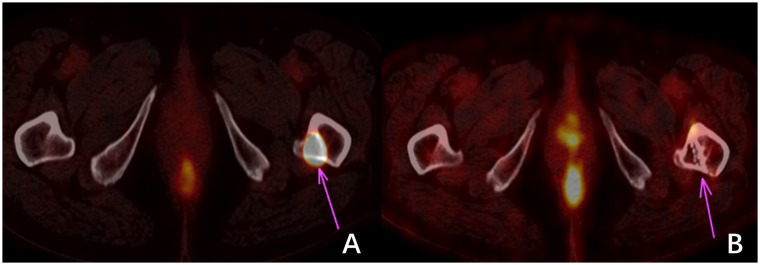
^18^F-FDG imaging of bone metastasis in the left proximal femur of thyroid carcinoma. (A) shows a high uptake of 18F-FDG in the metastatic focus before treatment. In contrast, (B) shows no significant change in the size of the metastatic focus and bone destruction three months after treatment. However, no 18F-FDG uptake indicates that the tumor cells were inactivated.
